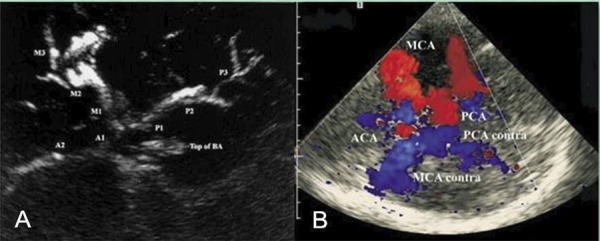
A. tUSA image of the circle of Willis and its branches after injection of ultrasound contrast agents. M1, M2, M3=middle cerebral artery segments; A1, A2=anterior cerebral artery segments; P1, P2, P3, P4=posterior cerebral artery segments; BS=brainstem; Top of BA=hyperechogenic distal part of the basilar artery. B. Contrast-enhanced transcranial ultrasound of the circle of Willis (axial scanning plane via the temporal bone window). Vessel delineation is diminished because of the strong contrast signal (“blooming”) after IV bolus injection of UCA. MCA=middle cerebral artery; MCA contra=middle cerebral artery contralateral; PCA=posterior cerebral artery; PCA contra=posterior cerebral artery contralateral; ACA=anterior cerebral artery.
